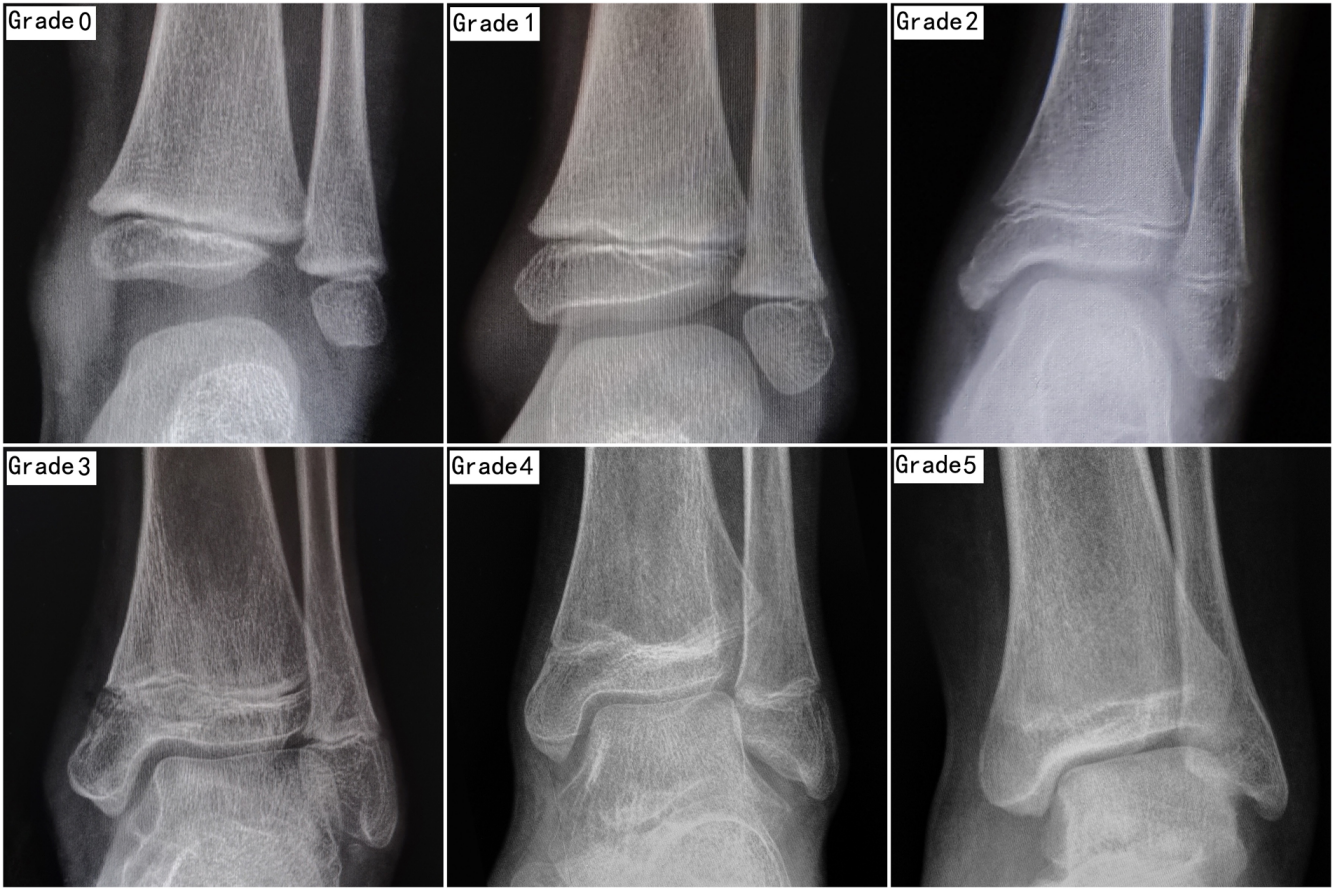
X-ray of grades of tibial epiphysis development.
